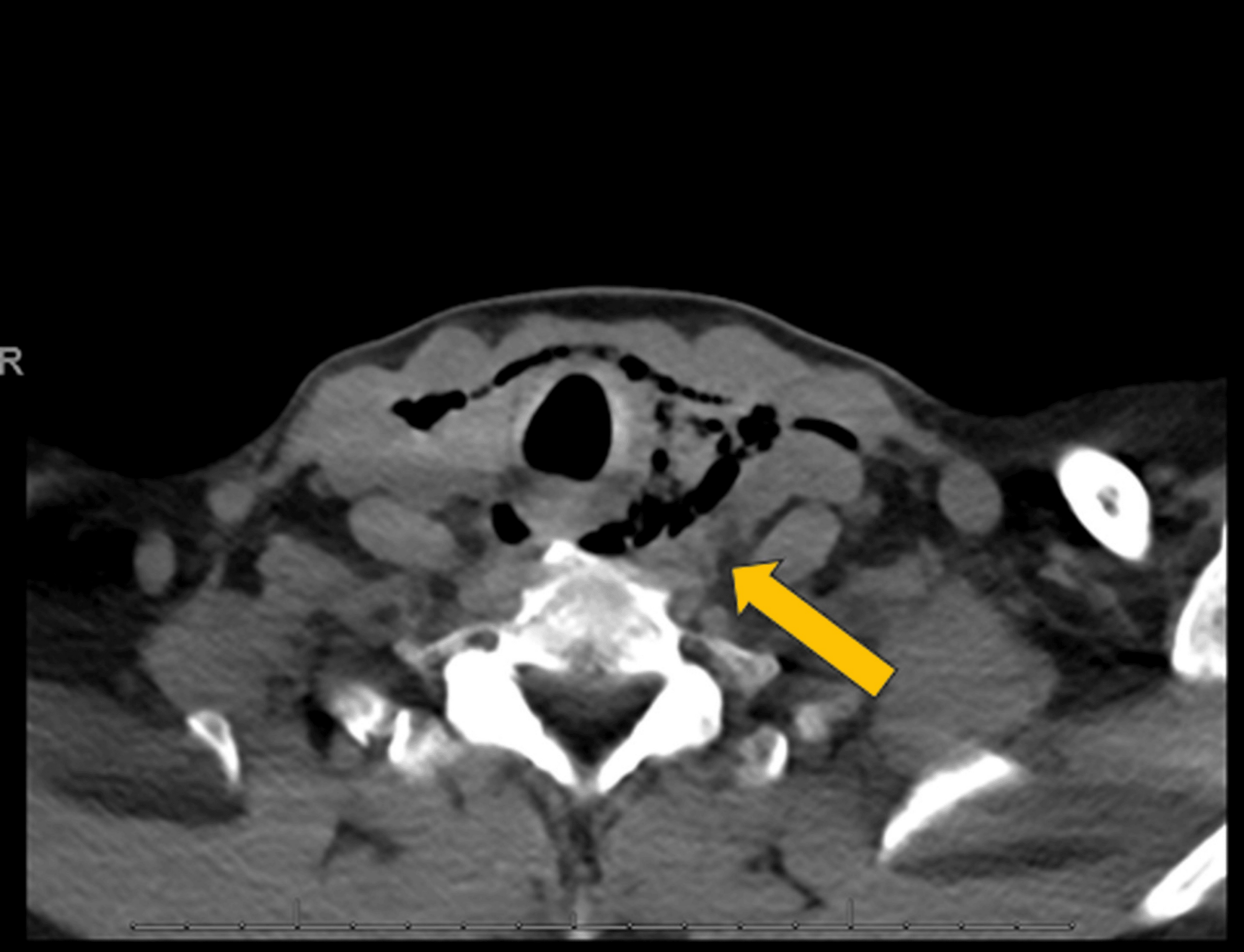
Computed tomography (CT) neck with oral contrast Extensive soft tissue air within neck with mild pneumomediastinum concerning for esophageal perforation.
